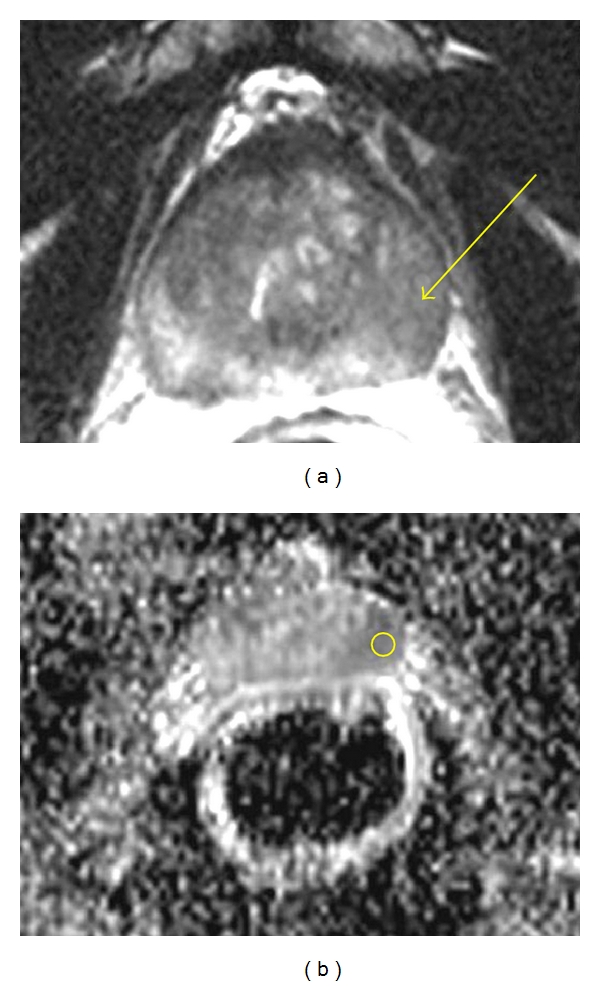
(a) T_2_-weighted MRI of 68 yo prostate cancer patient with GS 3 + 4 and (b) corresponding ADC map with low signal on the left base PZ.
